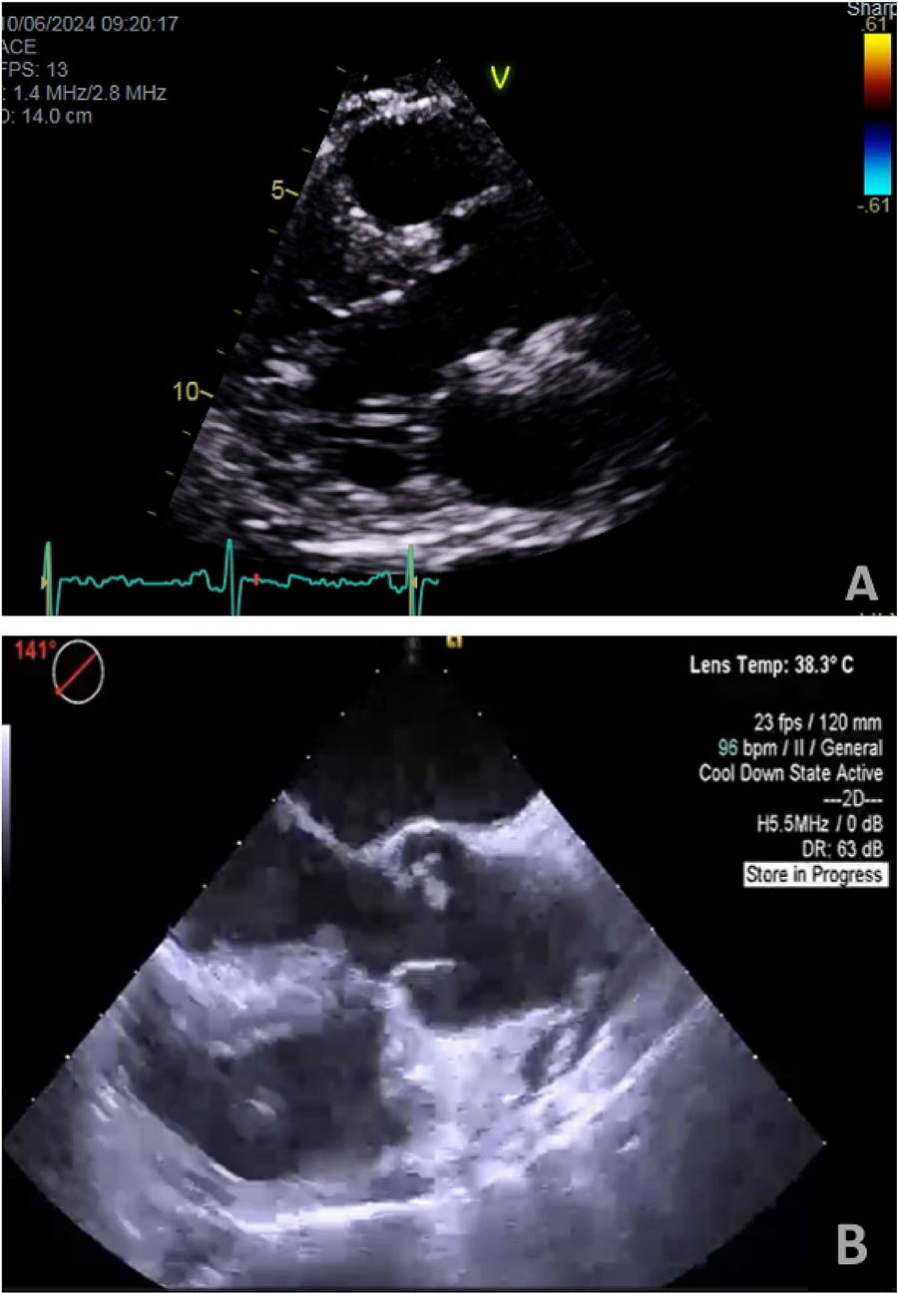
(A) Preoperative transthoracic echo (Para sternal long axis view) showing clear aortic valve cusps with no mass. (B) Intraoperative transesophageal echo (midesophageal long-axis view at 140°–150°) showing mass attached to ventricular surface of NCC of aortic valve.
